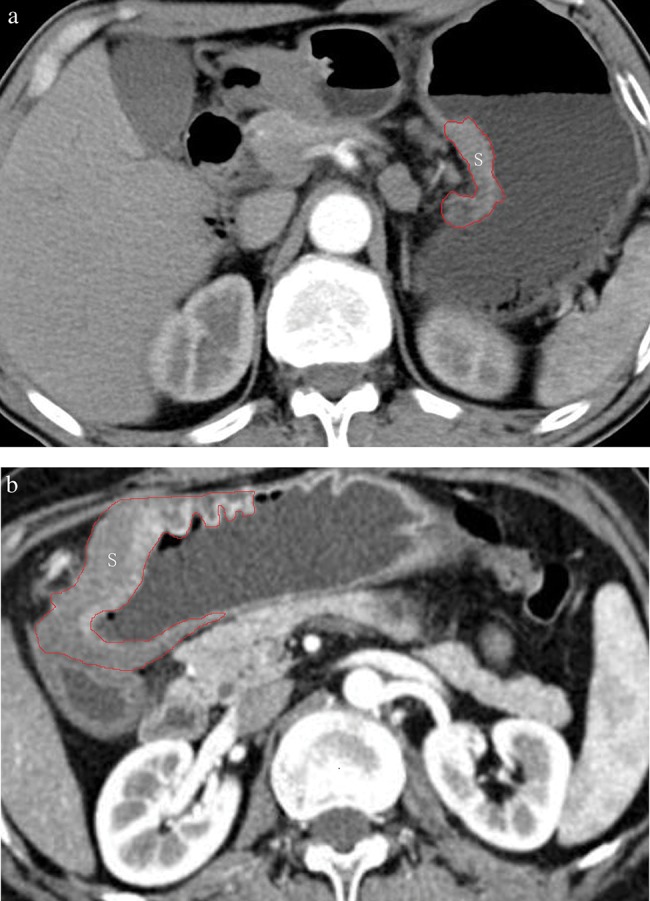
Transverse contrast-enhanced multidetector computed tomography (CT) images in a 55-year-old man (a) and a 65-year-old man (b) with gastric adenocarcinoma. The tumor area (S) is depicted on the axial contrast-enhanced CT image.
